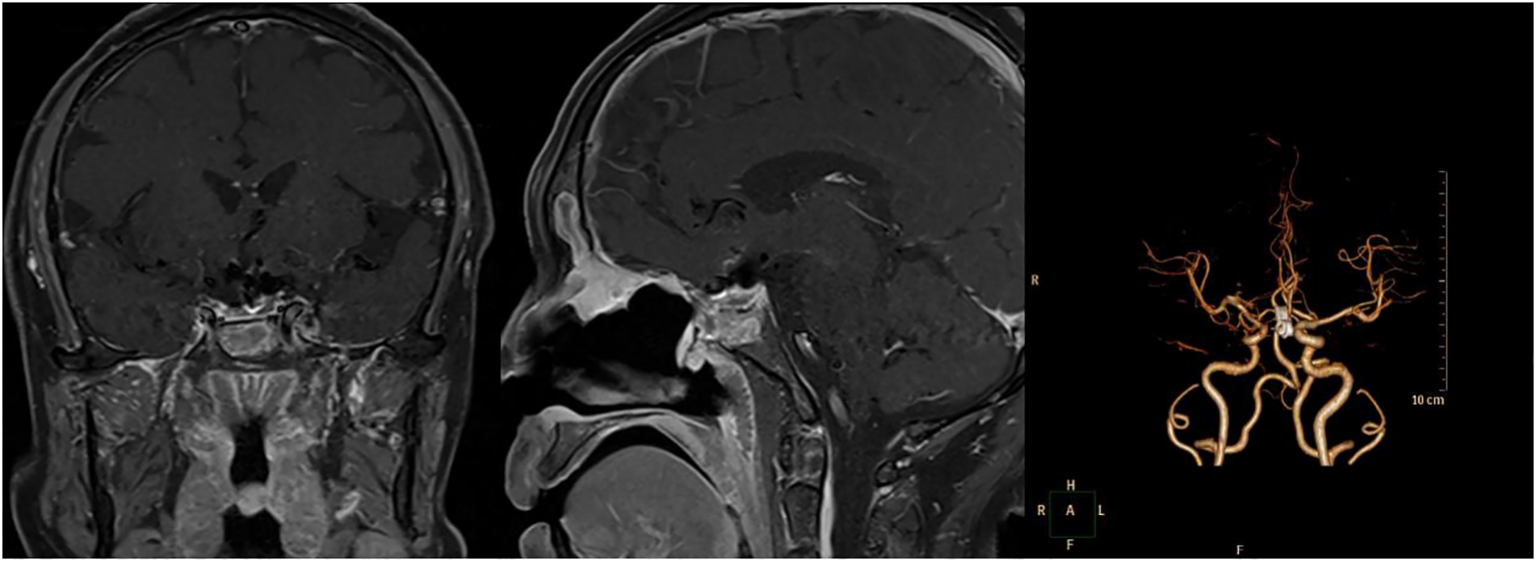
The detailed images reexamined 6 months after operation. Left and Middle: Pituitary enhanced MRI showed no recurrence of the pituitary adenoma 6 months after operation. Right: Computed Tomography Angiography (CTA) 6 months after operation showed the aneurysm was well clipped.
